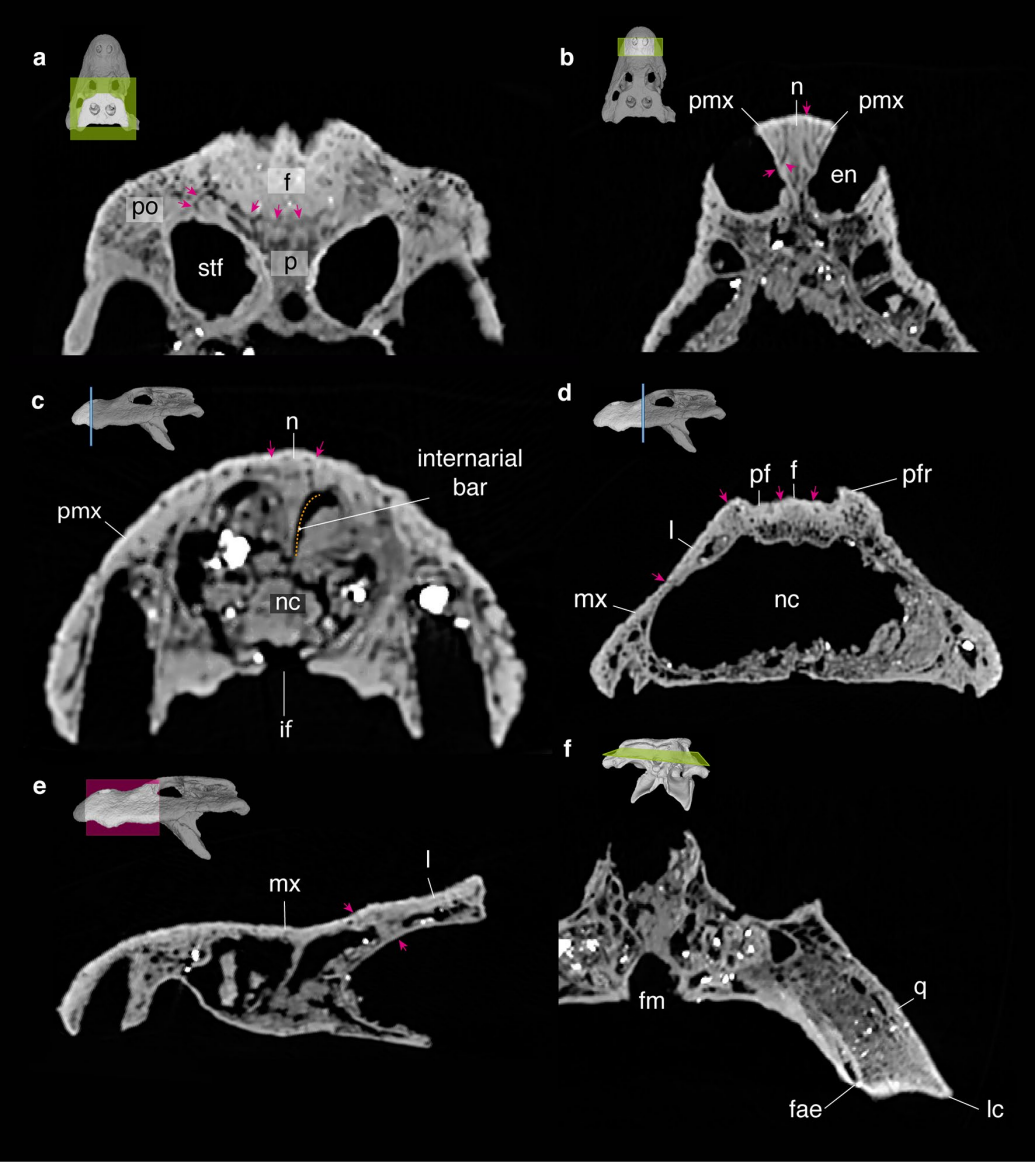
Selection of CT scan image stacks of *Alligator munensis* sp. nov. holotype (DMR-BSL-2011-2). Each figure component (a–f) is followed by the digitally reconstructed skull of *A. munensis* highlighting the area of interest. (a) axial cut of the skull table showing the fronto-parietal suture; (b) axial and (c) coronal cuts of the snout region showing the composition and morphology of the internarial bar; (d) coronal cut of the posterior portion of the nasal cavity; (e) sagittal cut of the antorbital region of the skull; (f) axial cut of the posterior position of the skull. Pink arrows indicate sutures between the bone elements. Dashed orange line indicates the contour of the internarial bar. *en* external nares, *f* frontal, *fae* foramen äerum, *fm* foramen magnum, *if* incisive foramen, *l* lacrimal, *lc* lateral quadrate hemicondyle, *mx* maxila, *n* nasal, *nc* nasal cavity, *p* parietal, *pf* prefrontal, *pfr* prefrontal ridge, *pmx* premaxilla; *po* postorbital, *q* quadrate, *stf* supratemporal fenestra.
